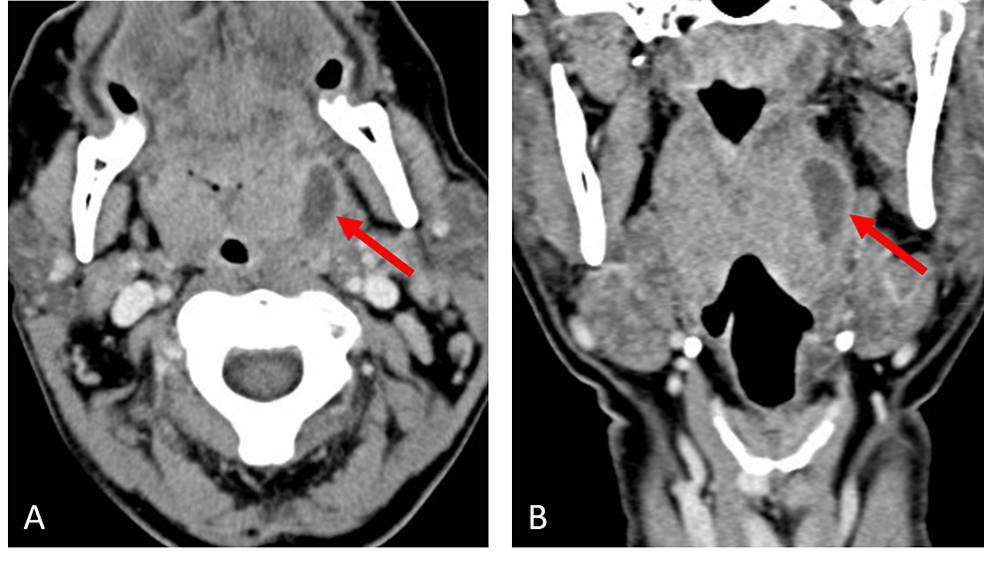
Peritonsillar Abscess Axial (A) and Coronal (B) View CT with contrast images demonstrating enlarged bilateral palatine tonsils and peripherally enhancing fluid collection in the left parapharyngeal space consistent with peritonsillar abscess (arrow).
